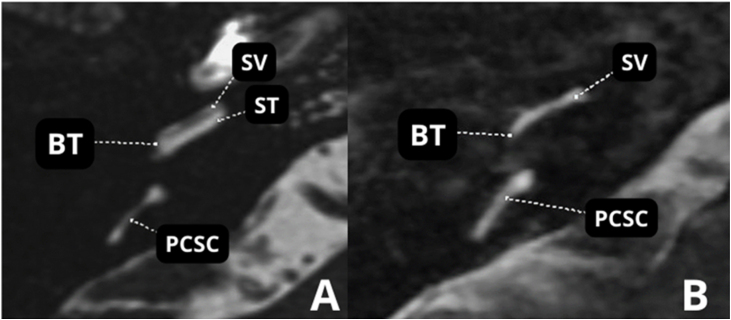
(A) MRI ‒ T2-weighted sequence A. Normal. (B) Advanced otosclerosis with showing stenosis of the scala tympani in the basal turn. BT, Basal Turn; SV, Scala Vestibuli; ST, Scala Tympani; PCSC, Posterior Semicircular Canal.
